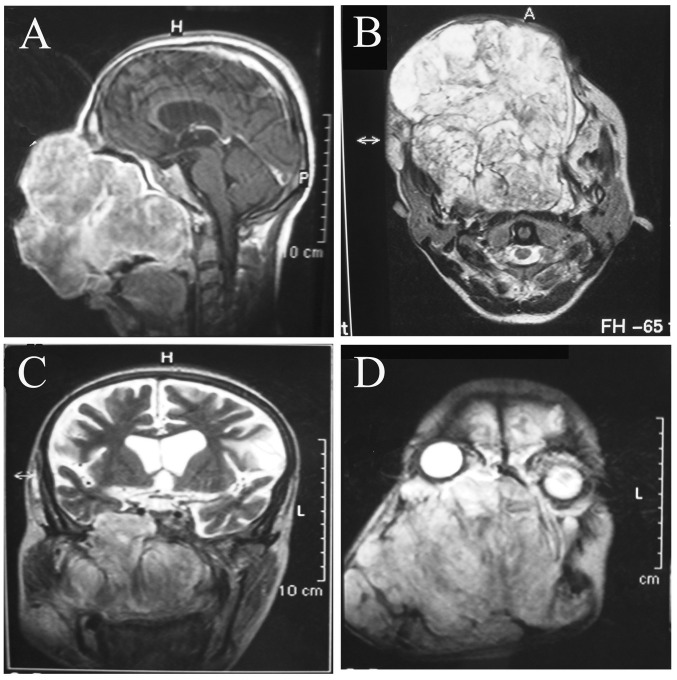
Patient with a large odontogenic myxoma of the partial left maxilla and whole right maxilla. (A) Sagittal T1-weighted MRI scan. (B) Axial T2-weighted MRI scan. (C and D) Coronal T2-weighted MRI scan revealing that the partial right sphenoid wing, the bilateral maxillary sinus and the nasal septum were absorbed by the tumor pressure. MRI, magnetic resonance imaging.
